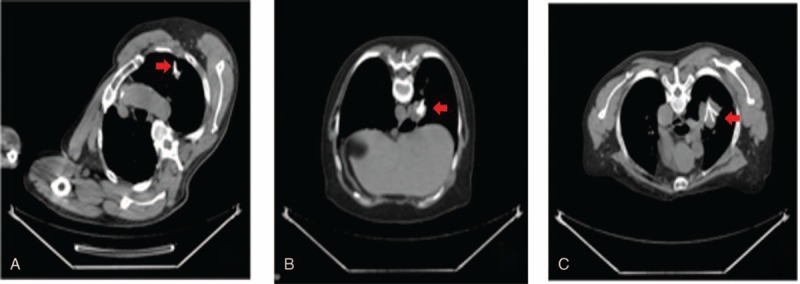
Placement of radiofrequency electrode was observed by a tiny, needle-like probe inserted to lung cancer tissues under CT guidance. Pretreatment CT scanning in patient 1 (A), 2 (B), and 3 (C) showed an active tip (red arrow) of a needle electrode within the targeted lesion. CT = computed tomography.
